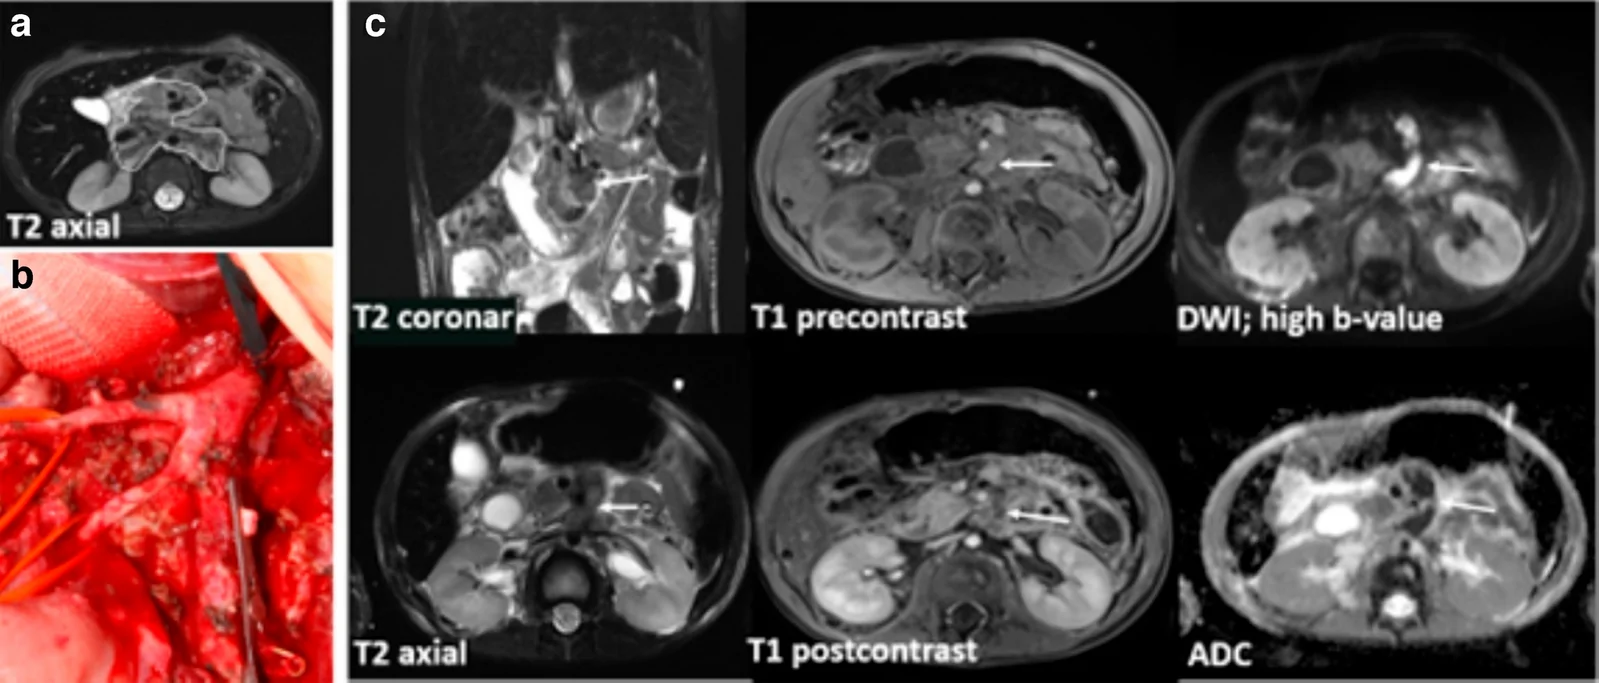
A 5-year-old female patient. a Preoperative magnetic resonance imaging performed 6 days after surgery shows a retroperitoneal neuroblastoma (*cursive*). b Intraoperative photograph demonstrates no visible residual disease in the resection area. c Early postoperative magnetic resonance imaging shows residual tumor adjacent to the duodenum (*arrows*). This discrepancy can be explained by the deep anatomical location of the tumor in the mesenteric root close to major vascular structures, limiting intraoperative visualization
